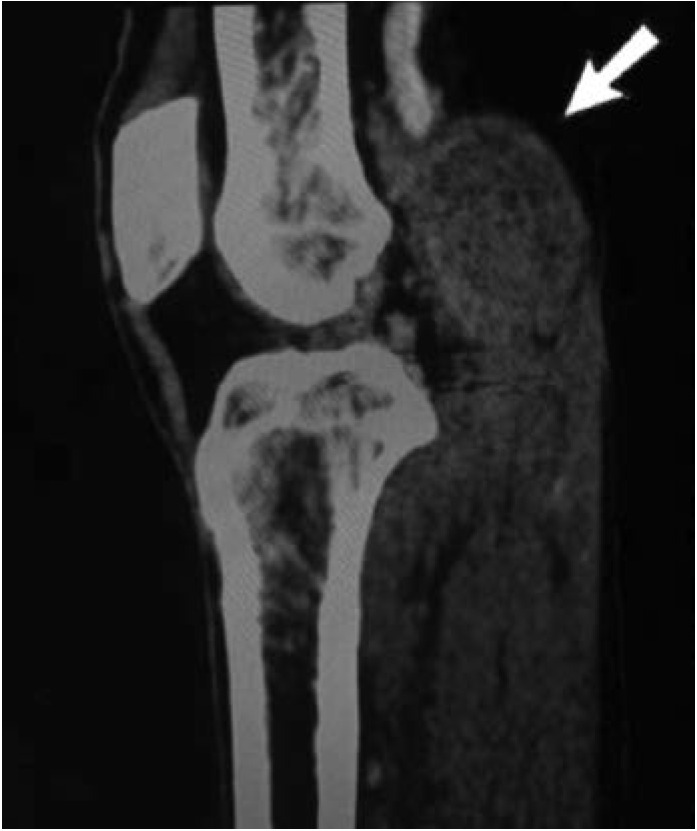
Contrast CT sagittal view showing a non-enhancing mass (arrow) posterior to the knee joint causing obstruction to popliteal artery.
